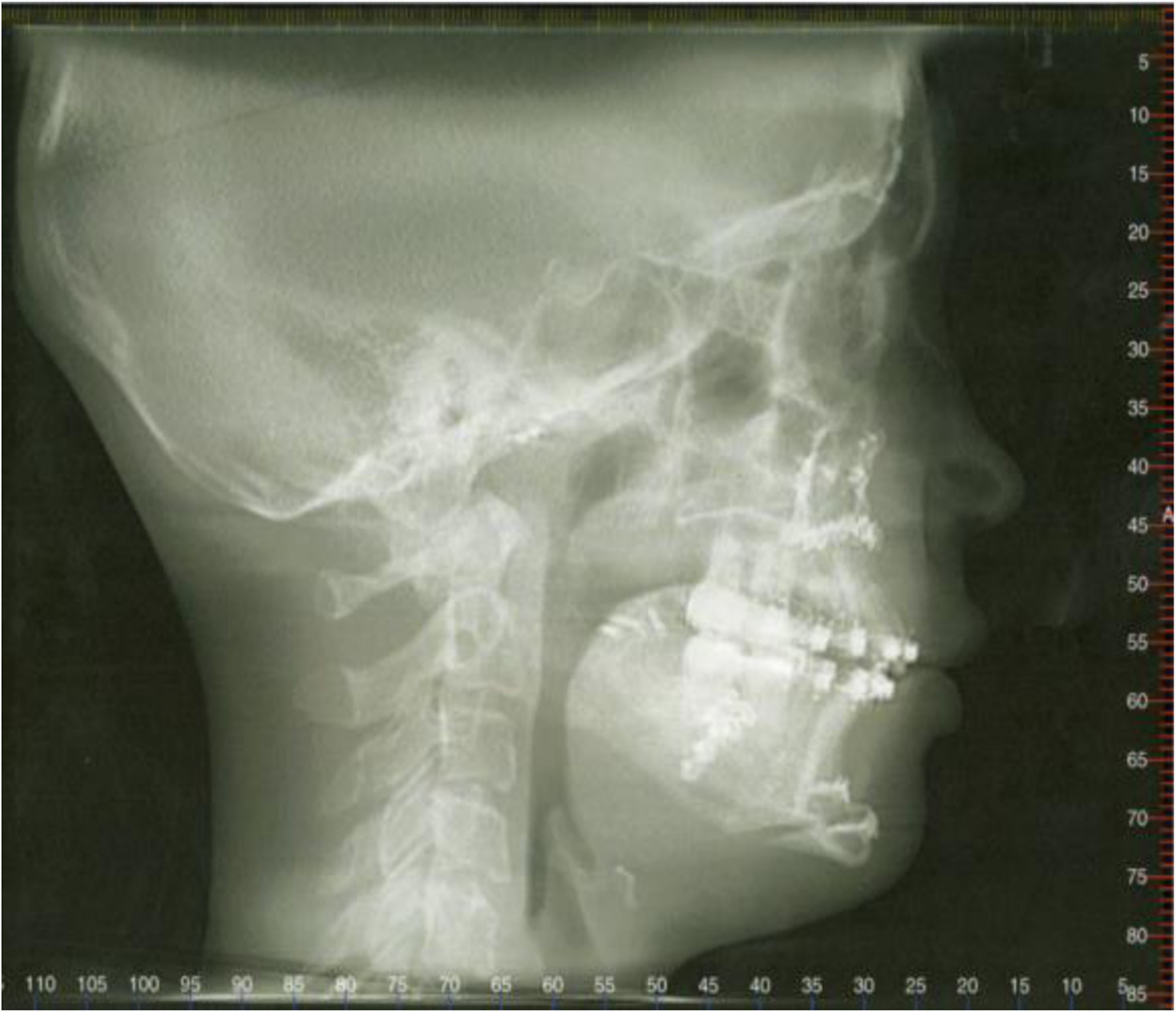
Post-surgical cephalometric radiograph demonstrating the rigid fixation devices in the proximal segment of the mandible used for superimposition
